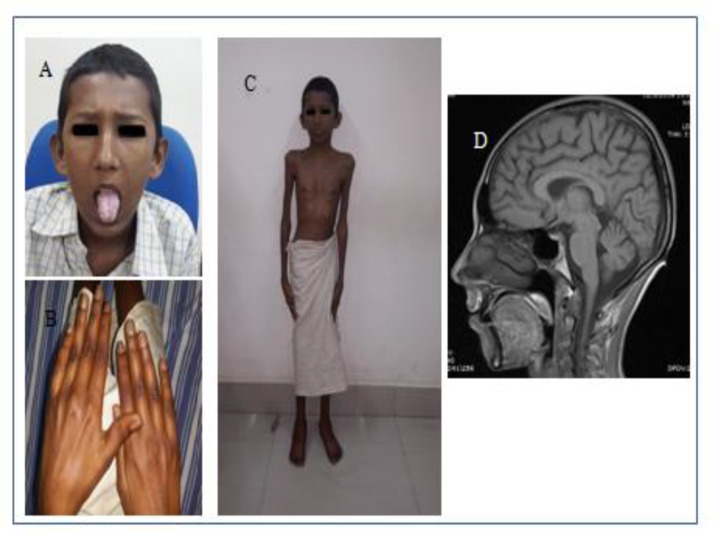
Image of the boy displaying the spastic atrophic tongue (A), hyperpigmented skin and thin wasted limbs and torso (B, C) and brain magnetic resonance imaging (MRI) T1W sagittal view showing pituitary hypoplasia (D)
